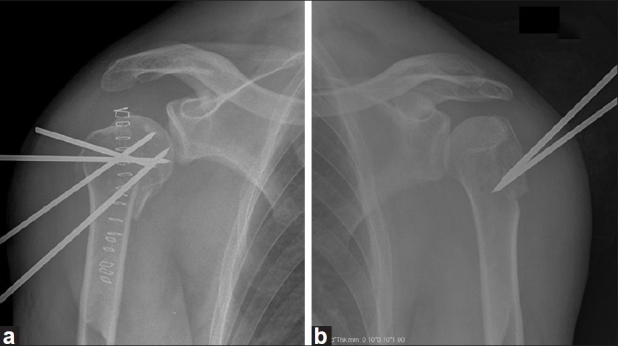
Radiographs (AP) of both [right- (a); left- (b)] shoulders 4 weeks after closed reduction under general anaesthesia and pin fixation with K-wires
